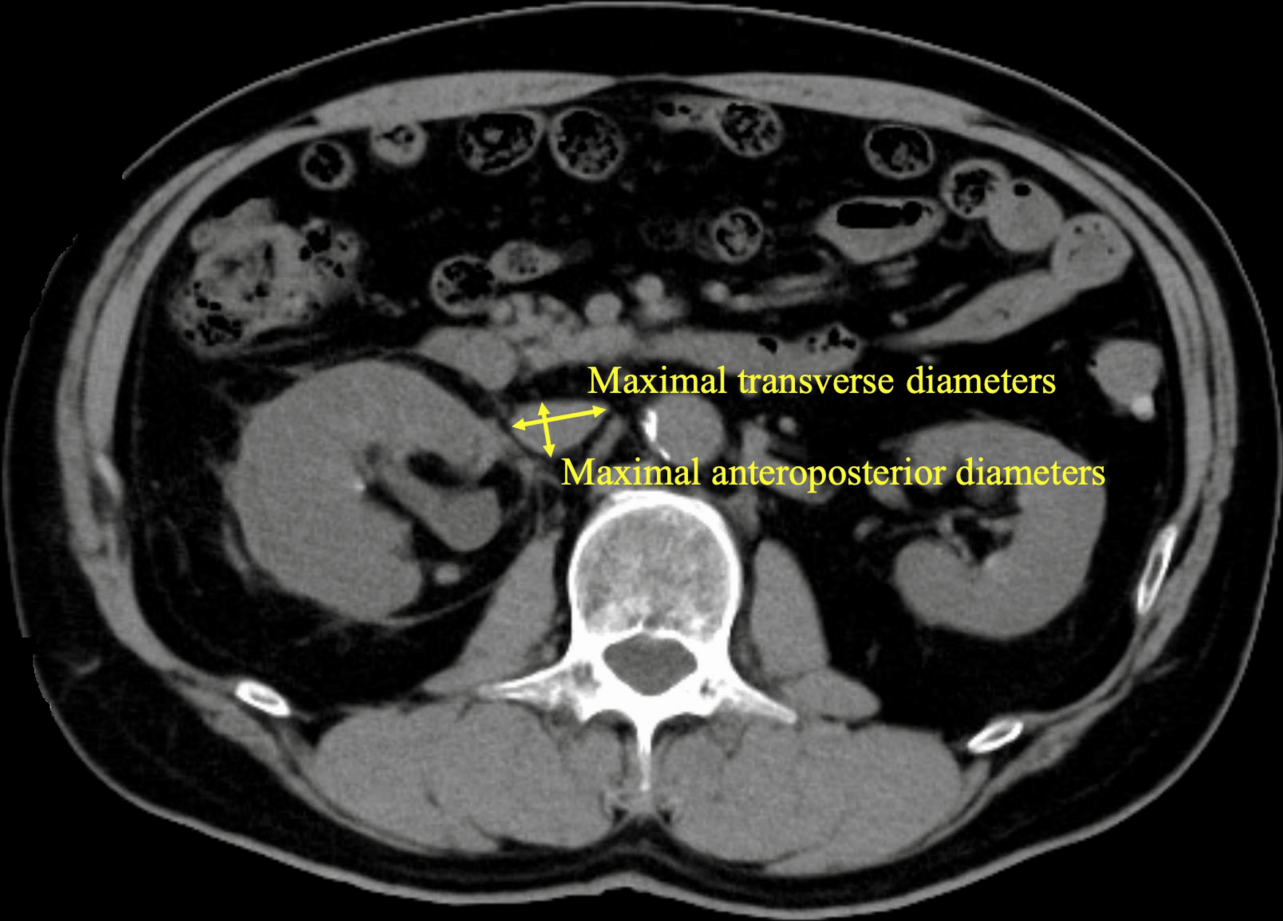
How to measure inferior vena cava (IVC) diameter in CT Measured on axial slice just inferior to the renal veins. AP/T=AP diameter/transverse diameter.
